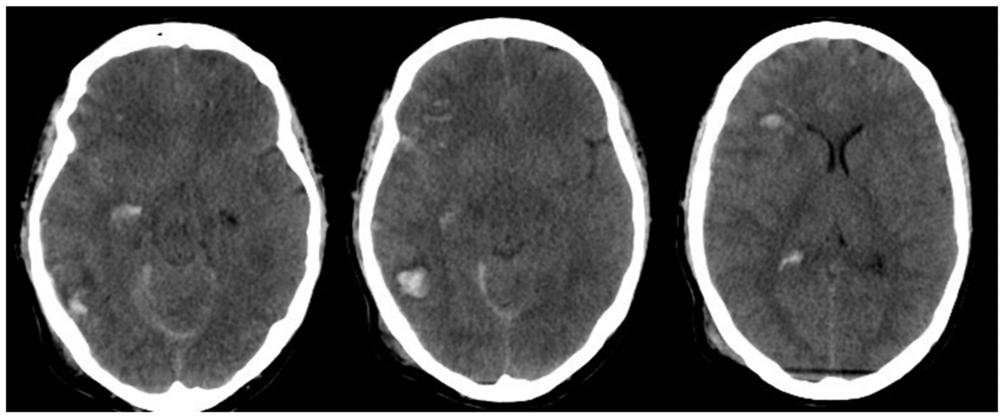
A fifty two year old female motorcyclist was involved in a motor vehicle accident. Initial Glasgow coma score was recorded as eleven (Eye: 3, Motor: 6, Verbal response: 2). Pupils were equal and reactive. She had sustained major pelvic and lower limb fractures. CT scan of the brain revealed diffuse petechial haemorrhages, traumatic subarachnoid haemorrhage, non evacuated haematoma and midline shift.
